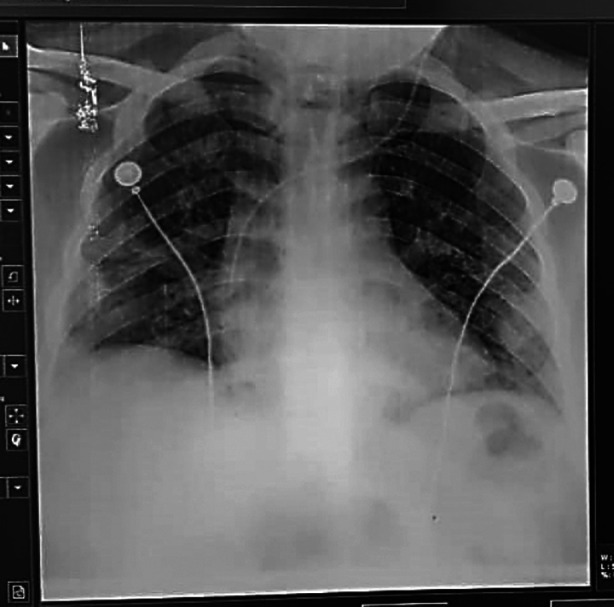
Same patient as in Fig 3(a) chest X-ray at discharge showed significant resolution of the opacities.
